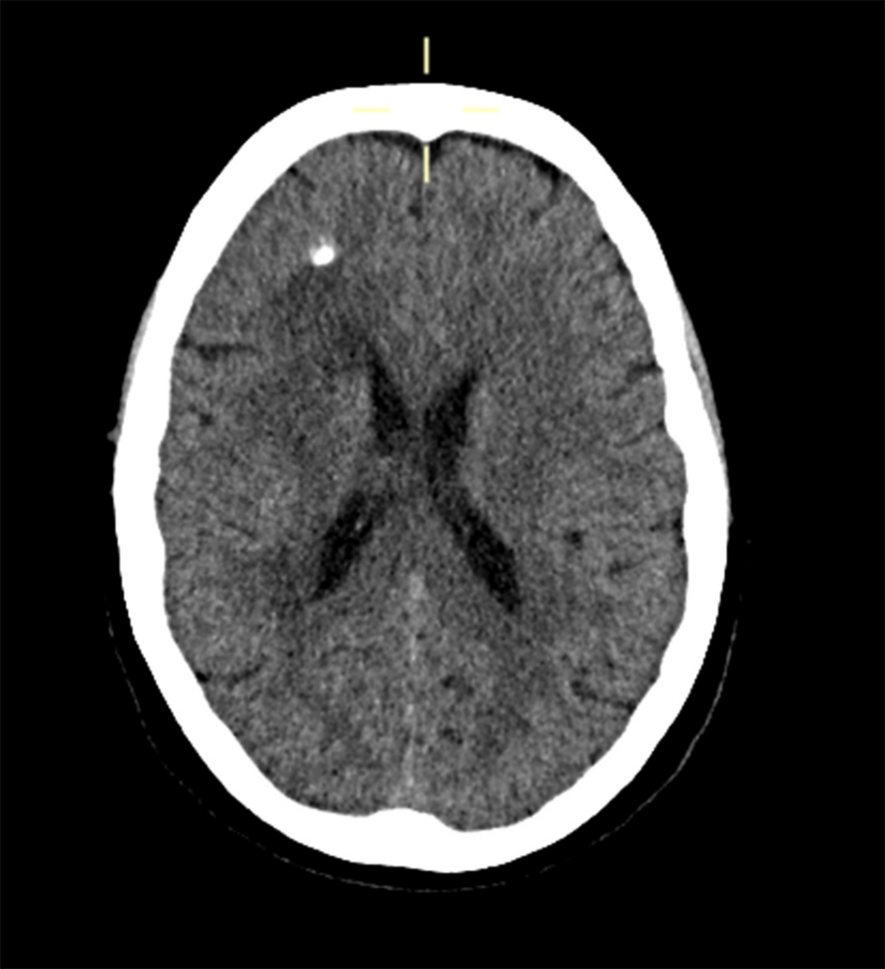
Unenhanced CT imaging of the brain from initial presentation showing a dense focus of calcification with surrounding low attenuation change.
